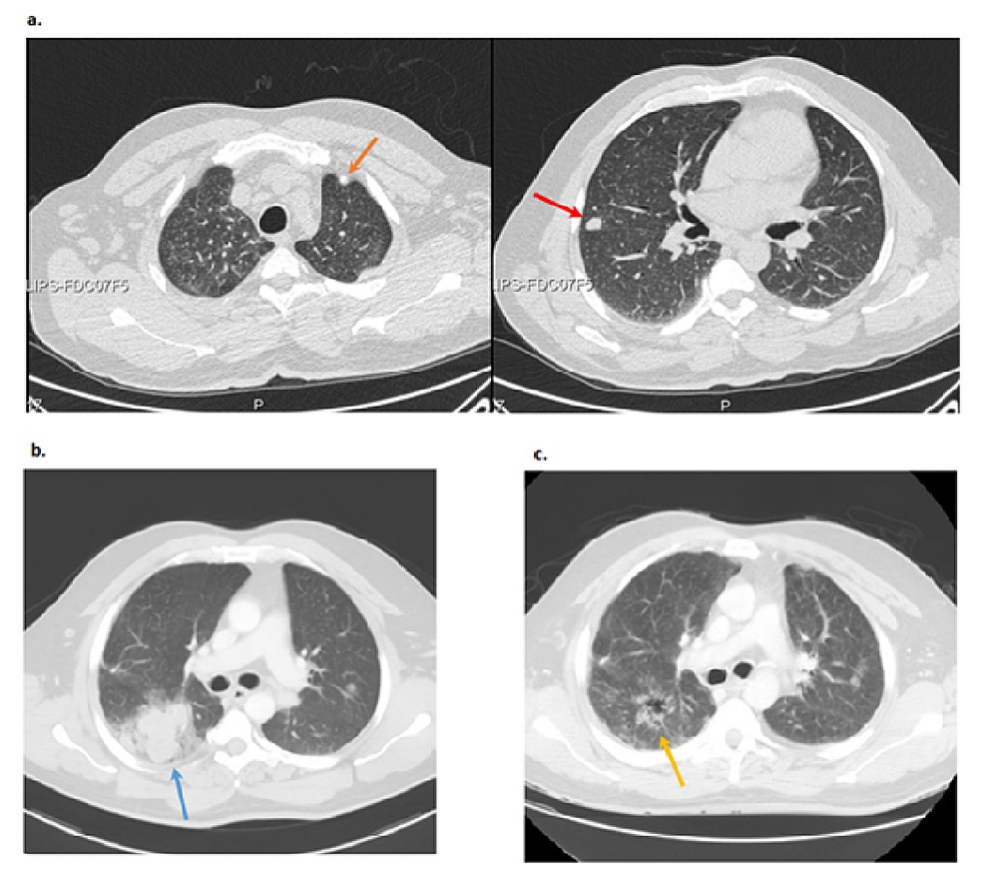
Evolution of LYG with lung involvement, before (a, b) and after treatment (c) a. Initial chest CT scan shows nodules in the anterior segment of the upper left lobe with 9mm diameter (orange arrow) and in the medium right with 12mm diameter (red arrow) b. Chest CT scan at six-month follow-up shows a localized mass in the apical segment of the inferior right lobe, with 55 x 39 mm (blue arrow) c. Chest CT scan at 18-month follow-up (six months after R-CHOP) shows regression of the mass previously seen in the inferior right lobe (yellow arrow) LYG: lymphomatoid granulomatosis; R-CHOP: rituximab, cyclophosphamide, doxorubicin, vincristine, and prednisone
